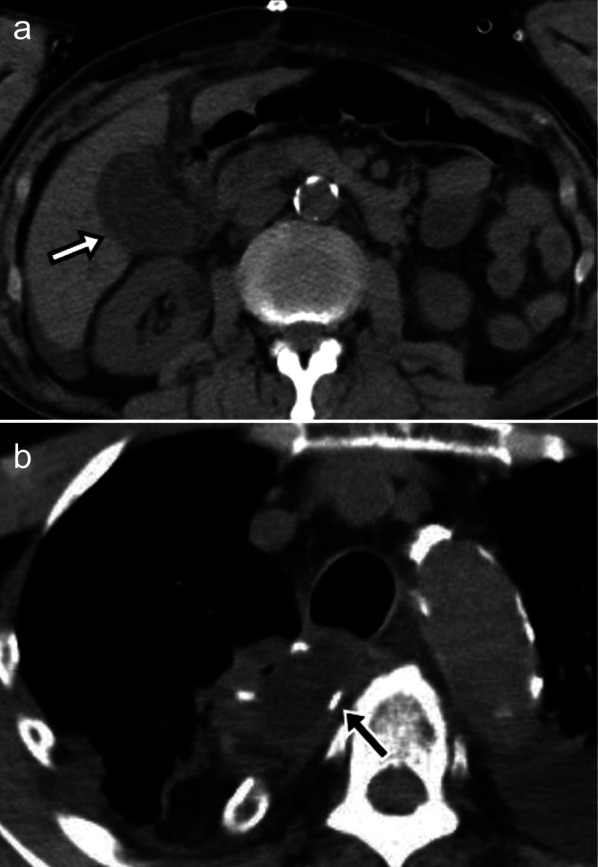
Computed tomography on the 4th postoperative day. a A medium-sized fluid collection is seen around the gallbladder (arrow). b No definite findings around the anastomotic site suggest anastomotic breakdown (arrow)
